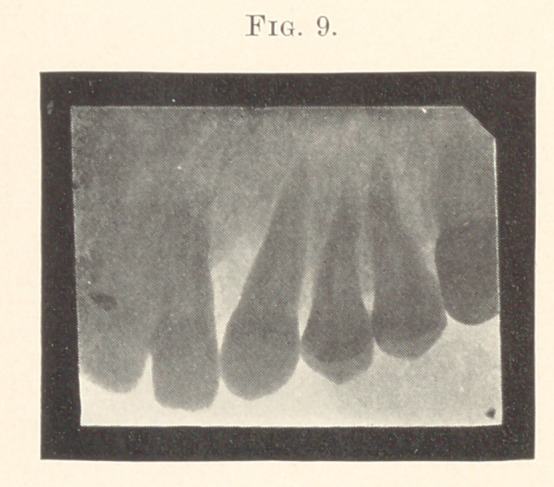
# A Case of Orthodontia Diagnosed and Progress Watched by the X-Ray
